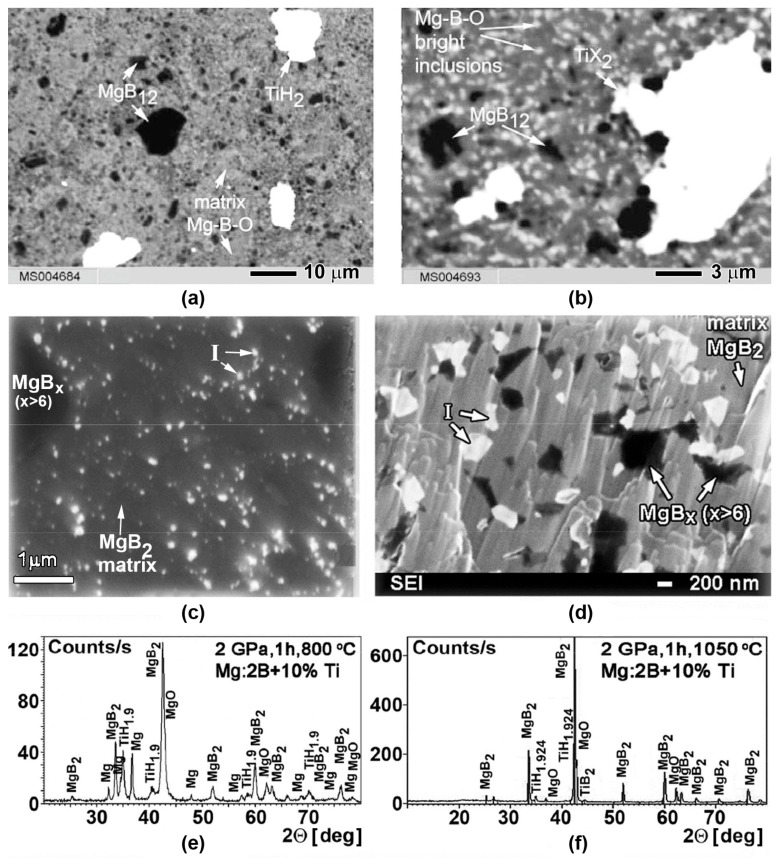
Microstructures of the materials synthesized from Mg(I):B(III) with a 10 wt% of Ti (3–10 μm) addition under 2 GPa for 1 h at 800 (a,c) and 1050 °C (b,d) [108]. X-ray patterns of these materials (e,f). (c,d) show the places where Ti is absent [103,113].
